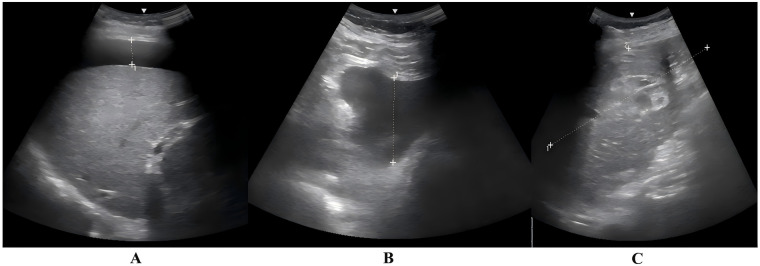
Bedside FAST exam findings. (A) Right upper quadrant ultrasound with free fluid surrounding the liver; (B) suprapubic ultrasound with free fluid in the abdominal cavity above the bladder; (C) left upper quadrant ultrasound with free fluid around the spleen.
